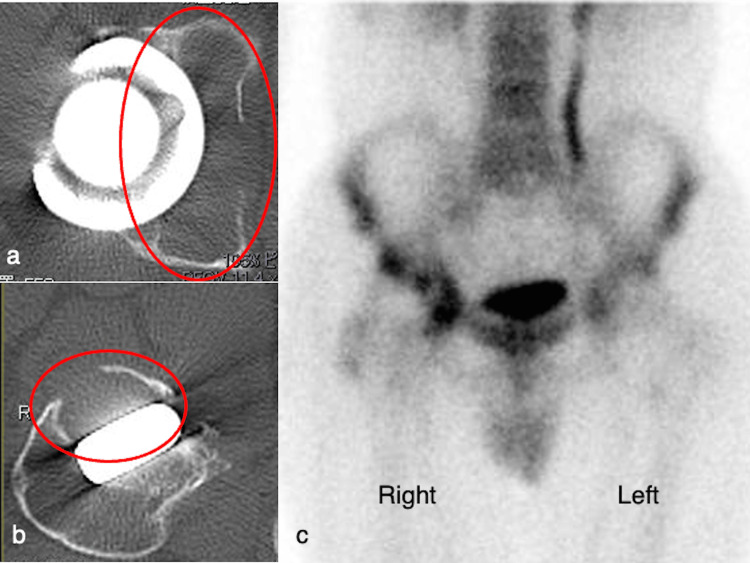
Plain computed tomography and bone scintigraphy findings. (a, b) The computed tomography images show severe osteolysis around the cup and stem; (c) the bone scintigraphy image shows uptake on the acetabular side.
